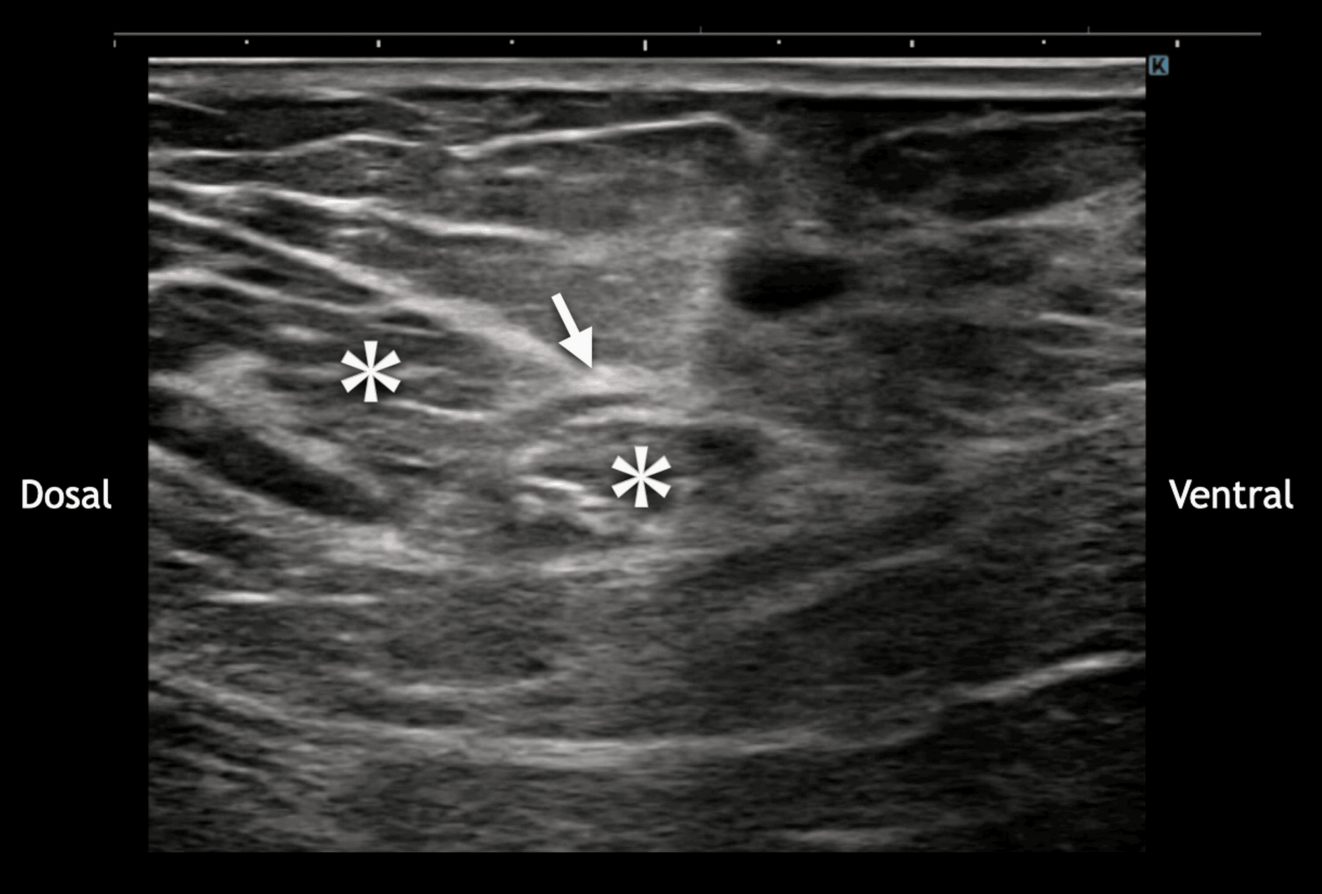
Short-axis ultrasound image of the sartorius muscle The infrapatellar branch of the saphenous nerve (IPBSN, arrow) penetrates the sartorius muscle (*). Localized tenderness was observed at this site, suggesting a potential entrapment point.
